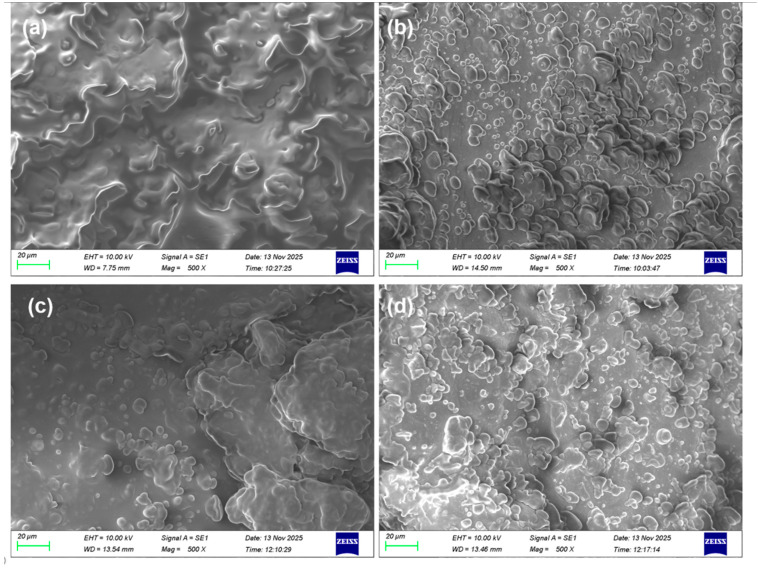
SEM of *perilla* seeds after different pretreatments: no ultrasound and ethanol pretreatment (a), ethanol addition without ultrasonic pretreatment (b), ultrasonic pretreatment without ethanol (c), and ultrasound–ethanol pretreatment (d).
